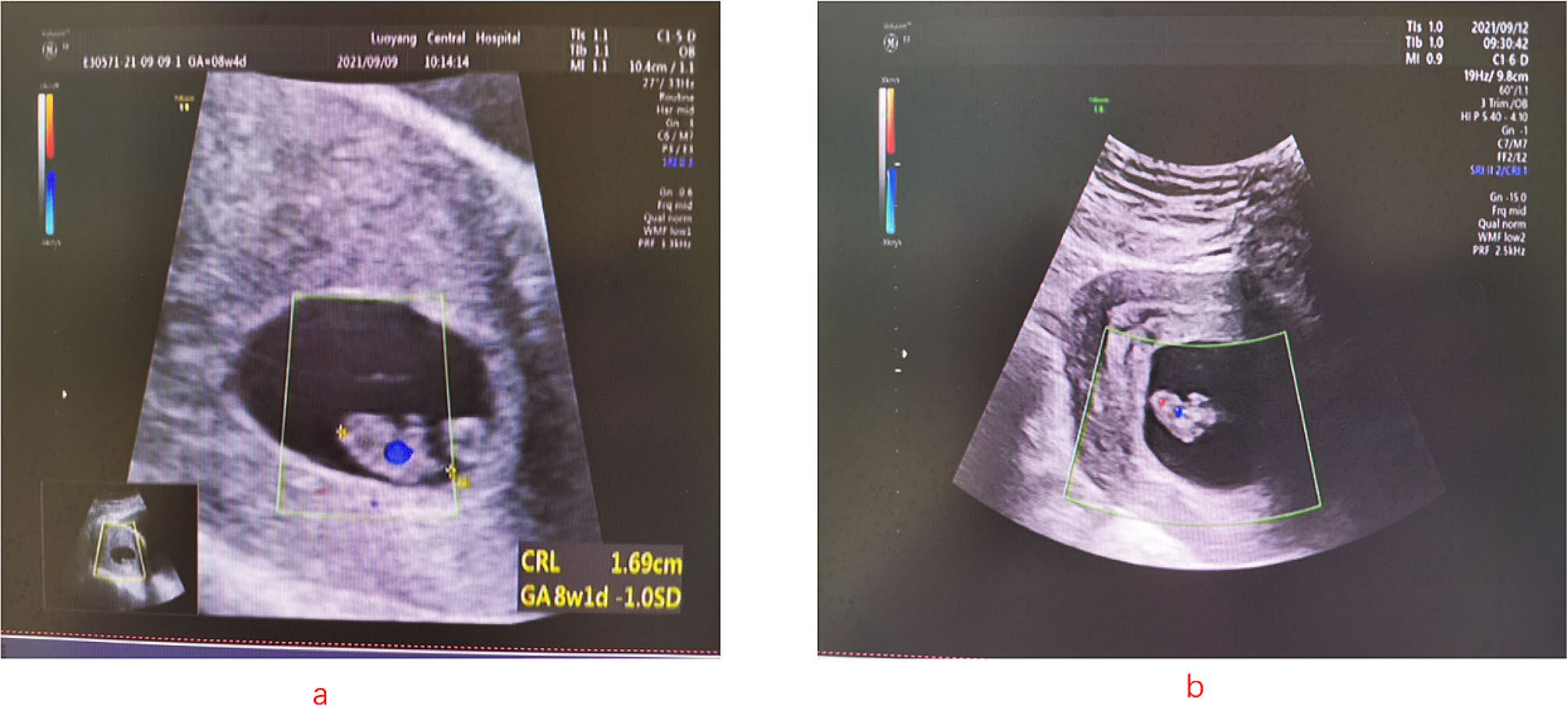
Figure (a) shows the ultrasound on the second postoperative day. Figure (b) shows the ultrasound on the fifth postoperative day. Both indicate intrauterine pregnancy
